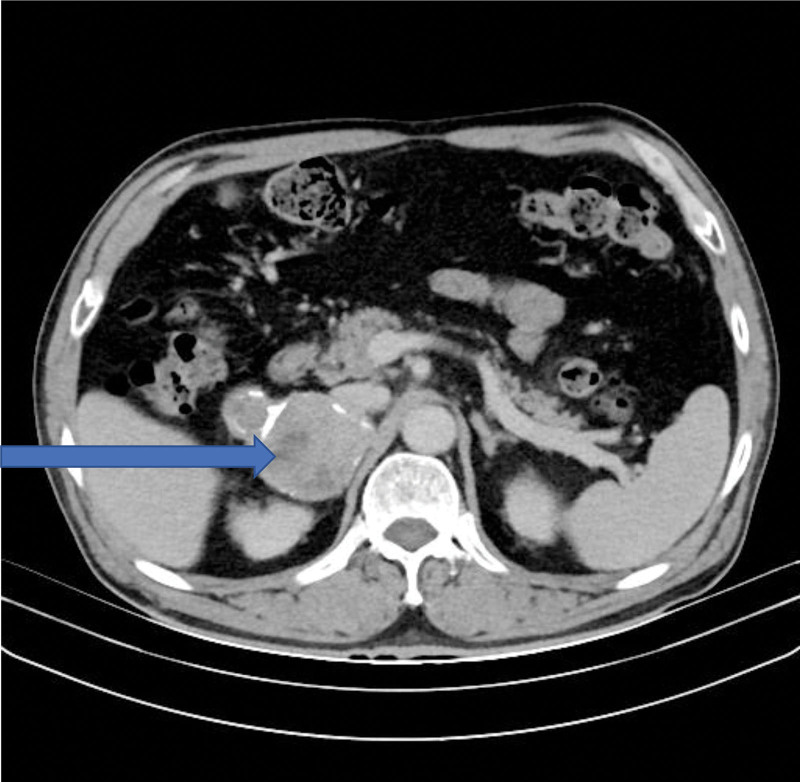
Abdominal contrast-enhanced CT image. Arrow indicates an irregular mass, measuring 5.0 × 4.8 cm, in the right adrenal gland, with progressive contrast enhancement. Nodular protrusions are evident at margin of the mass, which is surrounded by eggshell-like calcifications and bleeding is observed within it. CT = computed tomography.
